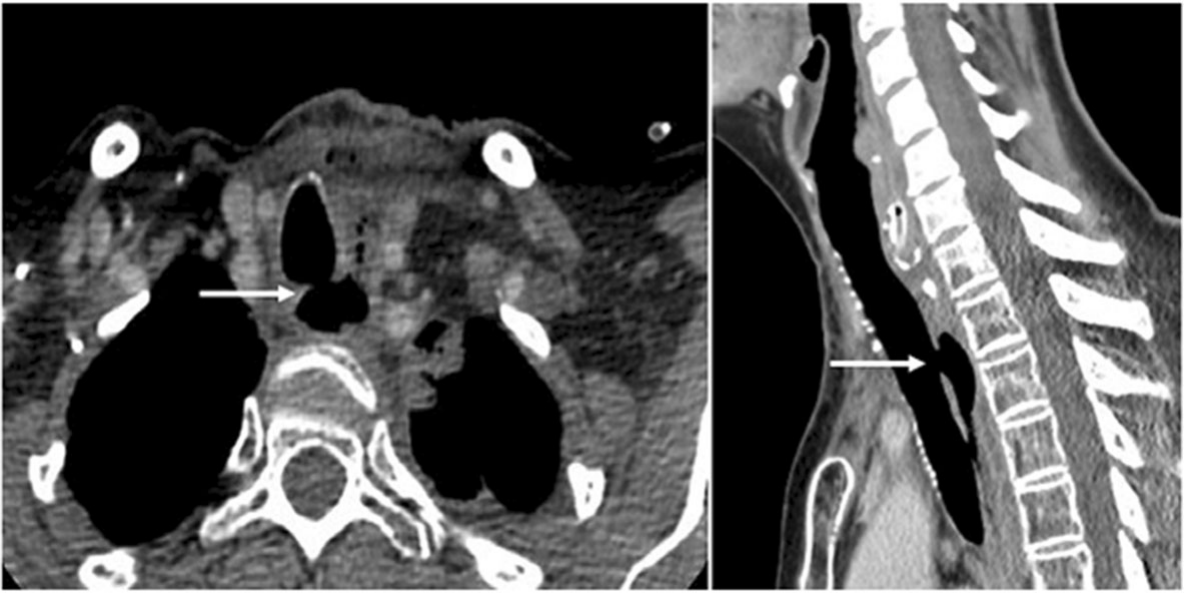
CT scan of Patient 1 demonstrating the aerodigestive fistula (arrows)
